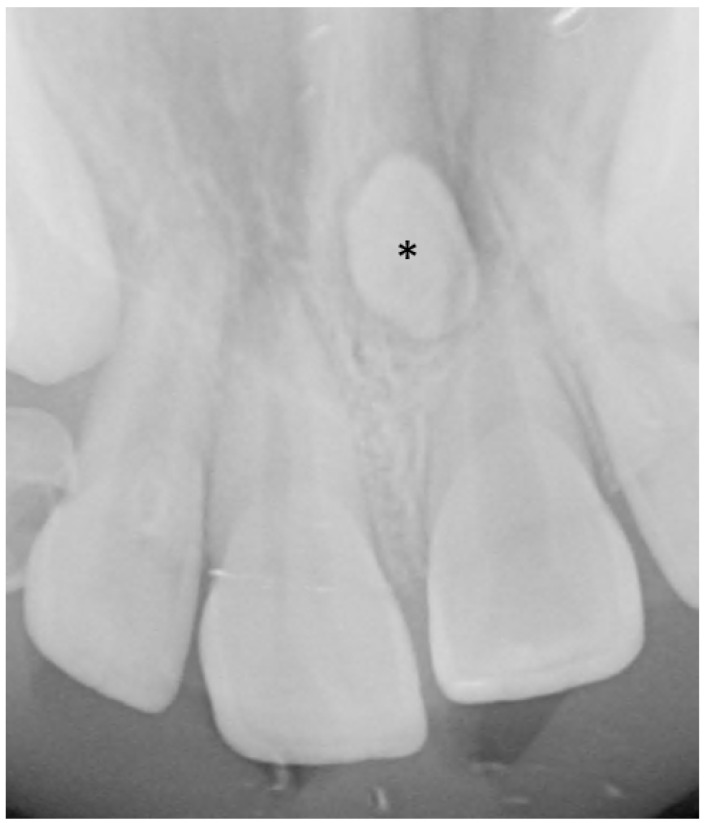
Supernumerary tooth (*) situated in between the roots of the central incisors, termed a mesiodens, shown on a dedicated dental radiograph.
